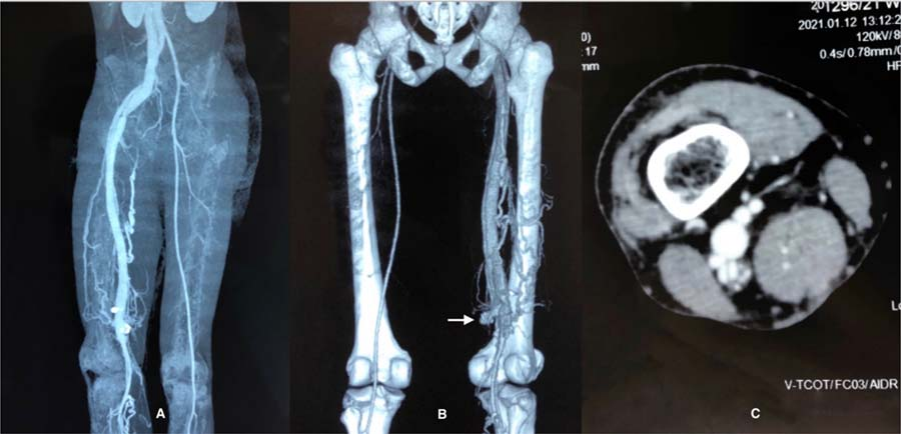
CT venogram (A) axial, (B) 3D reconstruction, (C) axial view at adductor cannel. There is early opacification of the popliteal vein in the arterial phase just below the level of adductor cannel on the right side and it is draining into inferior vena cava via the common femoral vein and iliac vein. The superficial venous system is not dilated. These features are consistent with high flow AVF most probably between the popliteal artery and vein.
